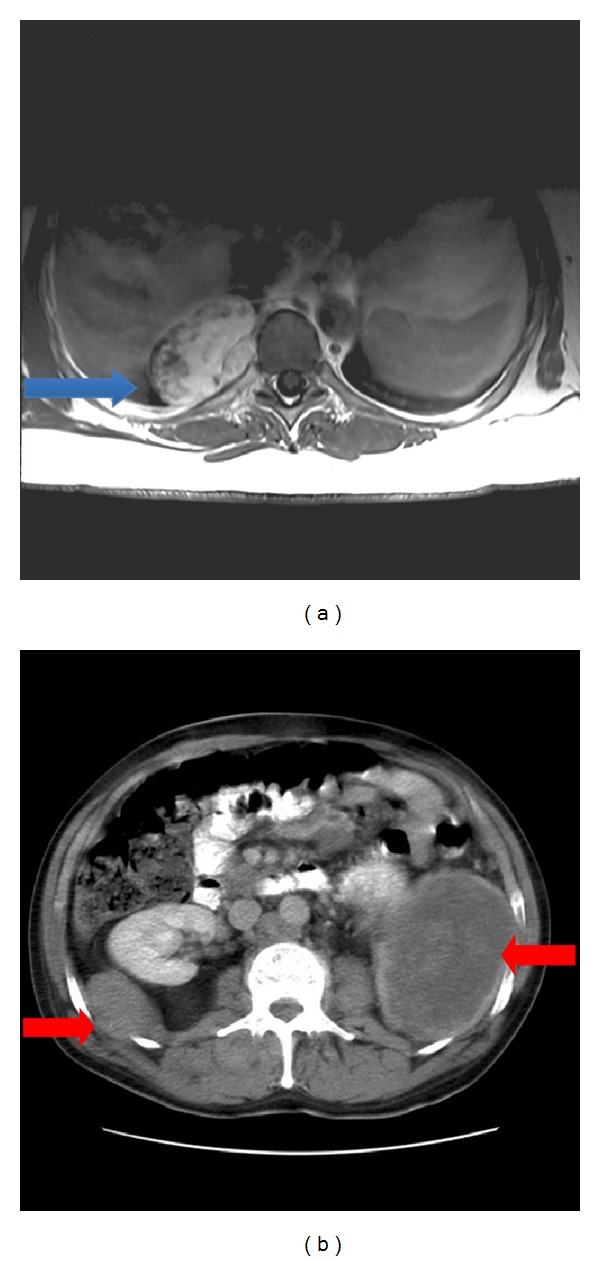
Magnetic resonance imaging showing a paravertebral metastasis of the thoracic cavity (blue arrow) just above the diaphragma diagnosed 12 years after operation for a primary tumour of the foot (and 6 months after a local relapse) (a). Abdominal computed tomography of a patient with primary tumour of the chest wall showing bilateral retroperitoneal metastases (red arrows) diagnosed at the same time as the primary tumour (b).
